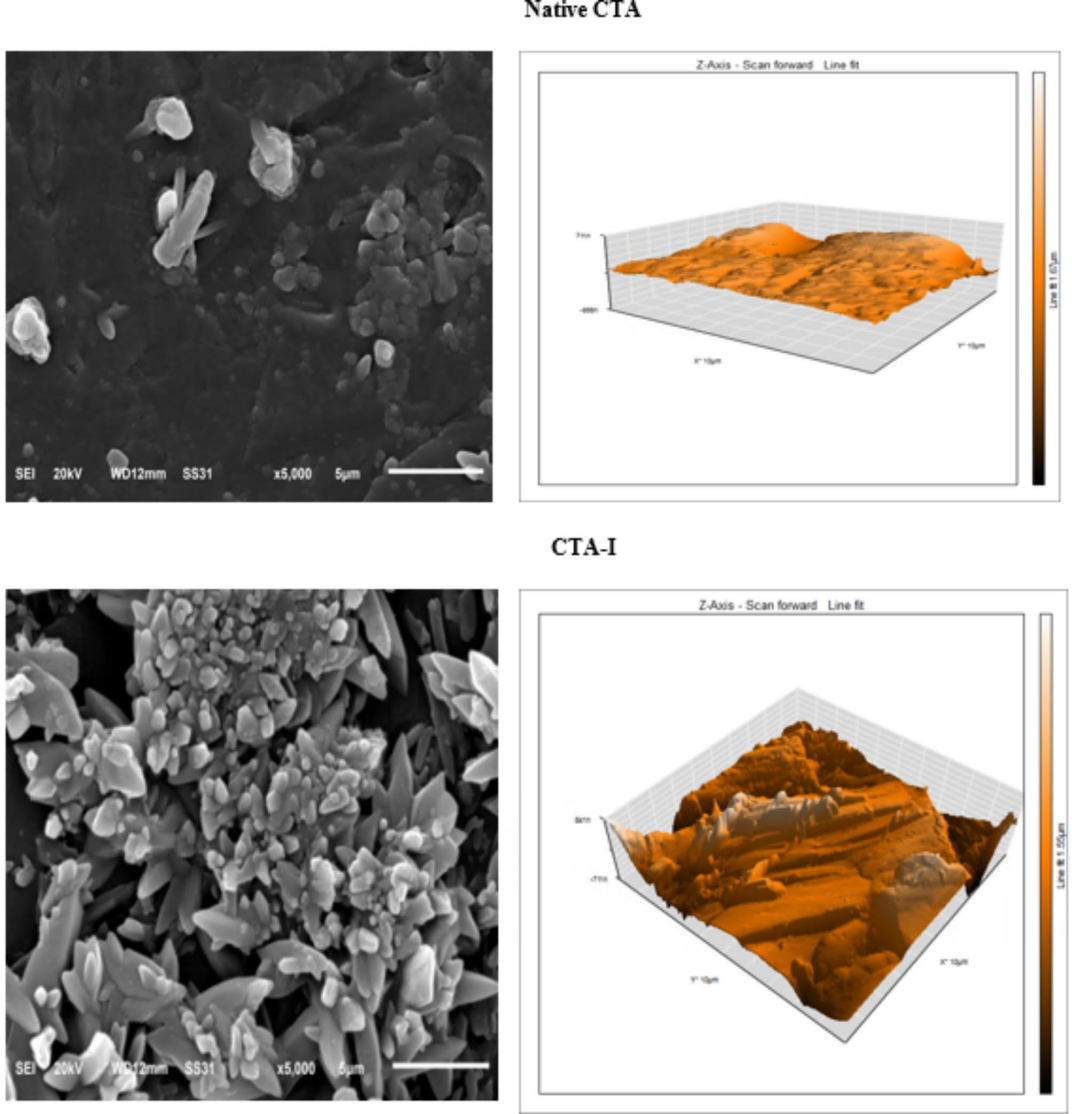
The SEM and AFM images of the native CTA, and CTA-I.
